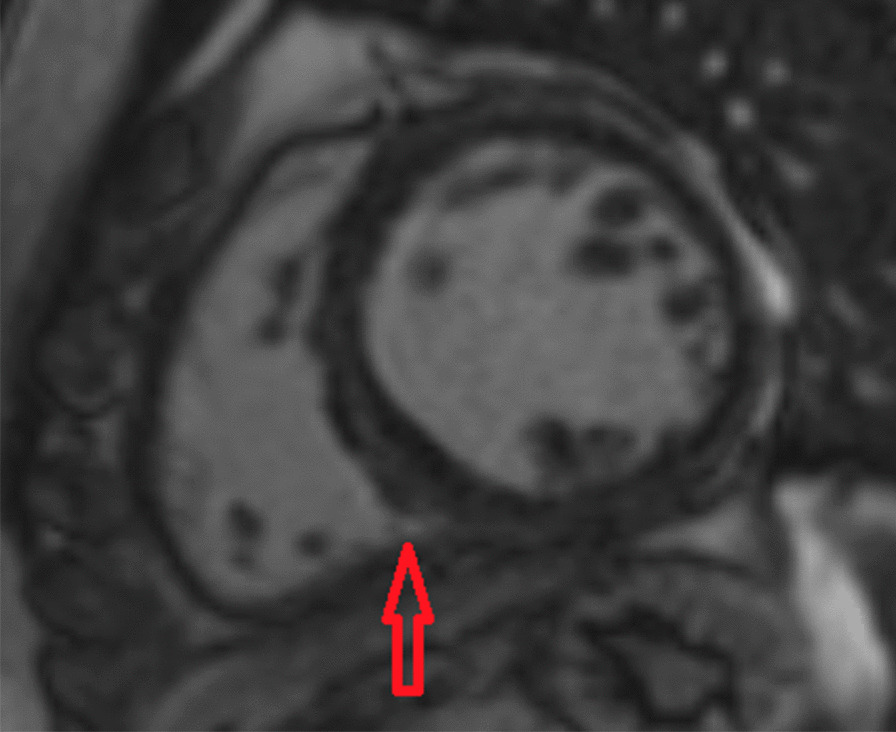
Cardiac magnetic resonance image in short axis view showing subepicardial late gadolinium enhancement in the interventricular septum suggesting previous myocarditis
